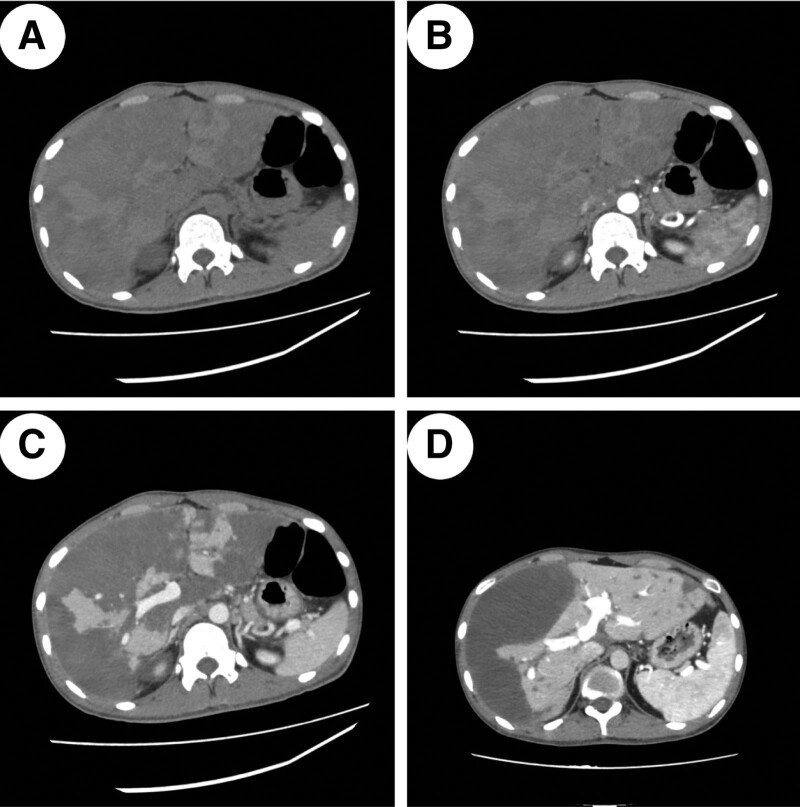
Hypereosinophilic Syndrome with extensive hepatic infarction. (A–C) Sagittal CT images showing plain scan, arterial phase enhanced scan, and portal venous phase enhanced scan, respectively. (D) Follow-up portal venous phase enhanced CT scan 2 months later.
